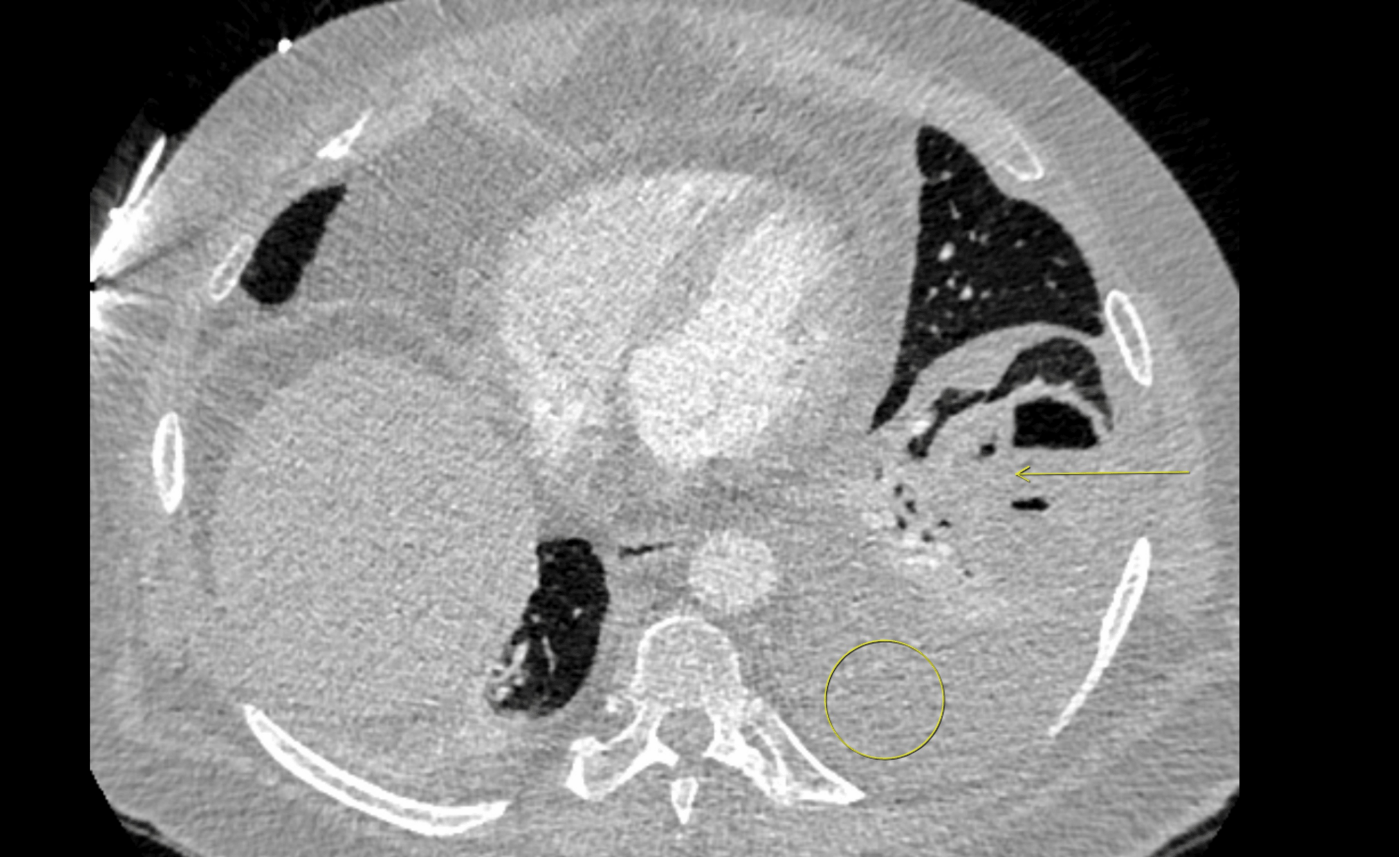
Axial CTA chest lung window two months prior showing left lower lobe consolidation with an abscess cavity (arrow) and moderate left pleural effusion (circle) CTA, computed tomography with angiography
